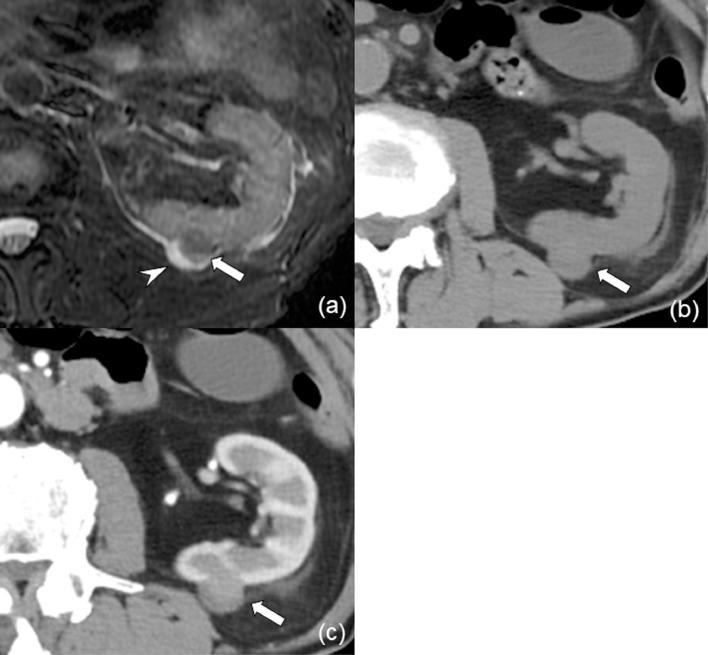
Images of the renal mass before initial cryoablation. (A) Fat suppression T2-weighted magnetic resonance image shows a homogeneous hypointense mass (arrow) on the dorsal border of the left kidney. The mass is adjacent to a renal cyst (arrowhead). (B, C) Computed tomography (CT) images of the mass. Contrast-enhanced CT image shows lower contrast enhancement of the mass (arrow) compared with the normal renal cortex in the corticomedullary phase.
